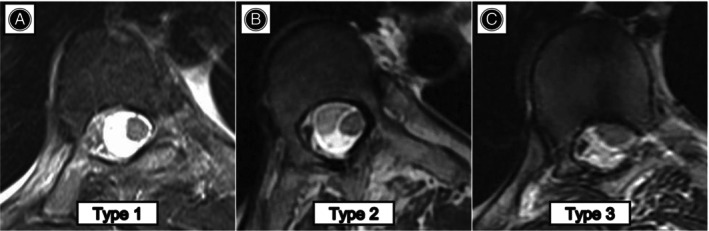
Representative images of spinal cord classification based on MRI.
